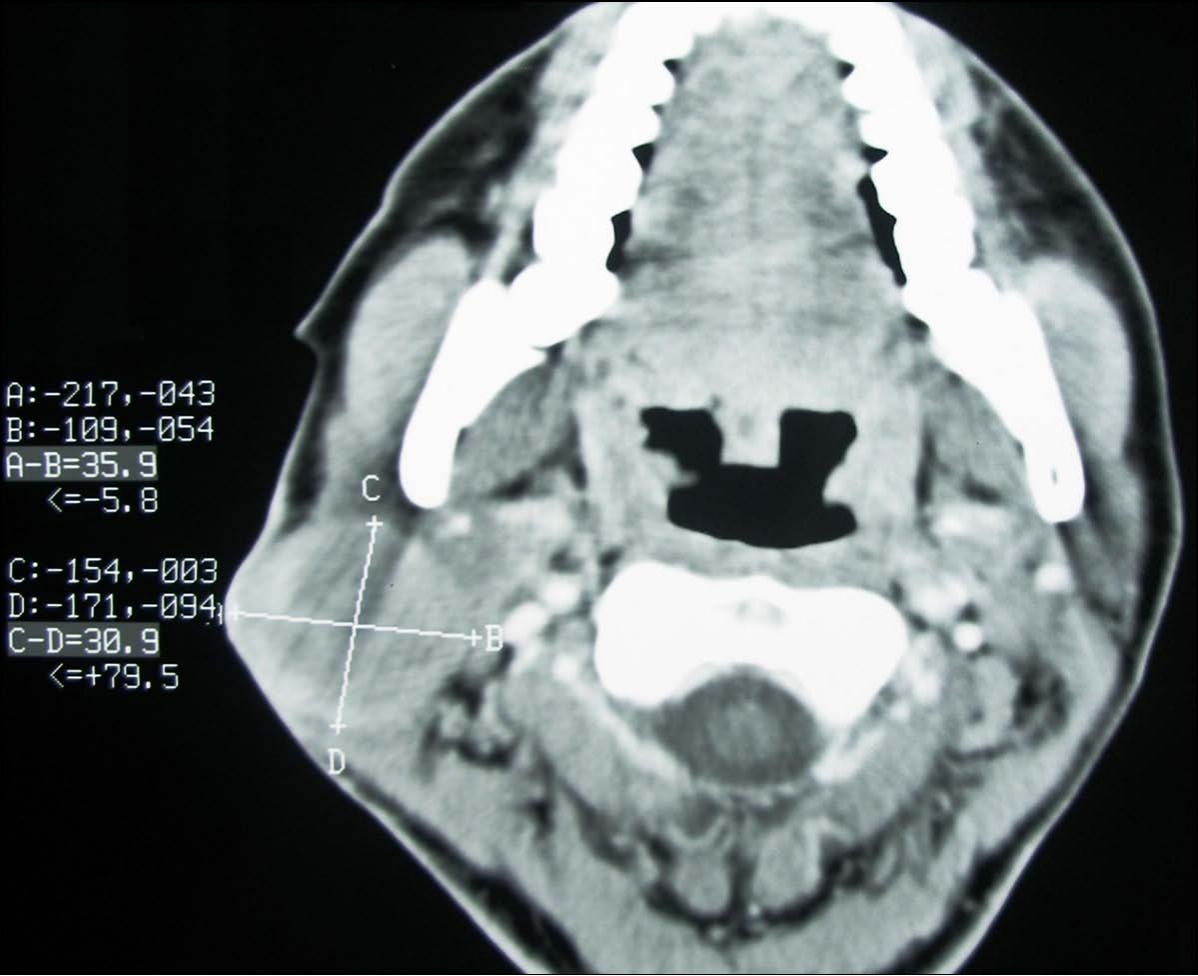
Computed tomography image showing a parotid mass adherent to overlying skin.
